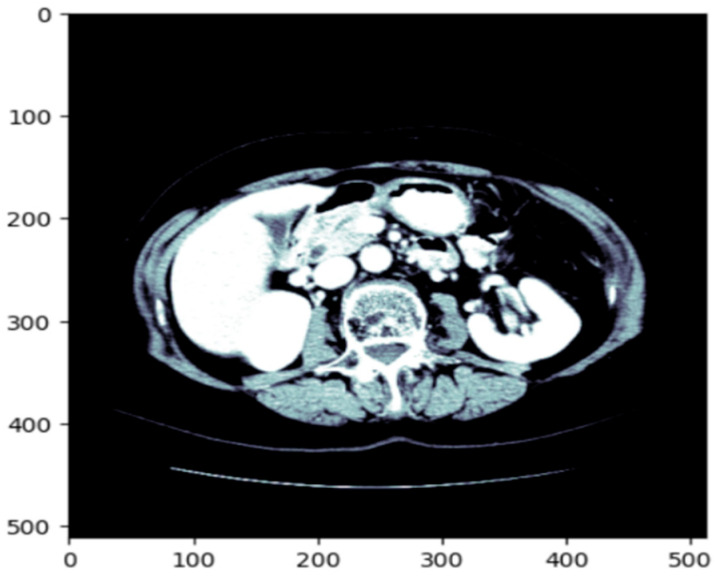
The original CT slice which enhances the contrast of the liver region to improve segmentation accuracy.
